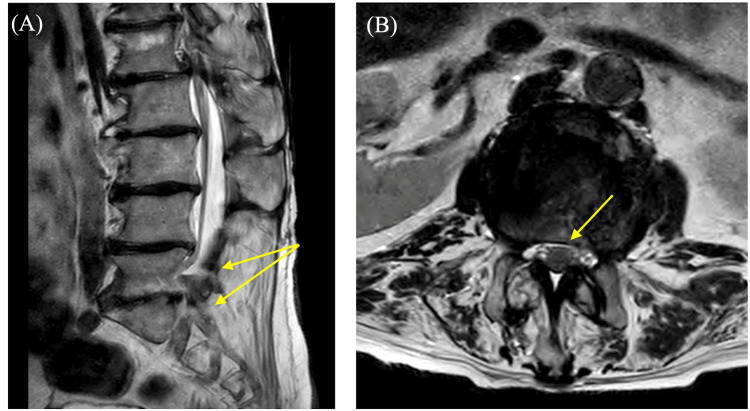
Magnetic resonance imaging of the lumbar spine (A) Sagittal and (B) axial views show severe lumbar spinal stenosis, hypertrophy of the ligamentum flavum, and compression of the thecal sac and cauda equina structures at the L5-S1 level.
